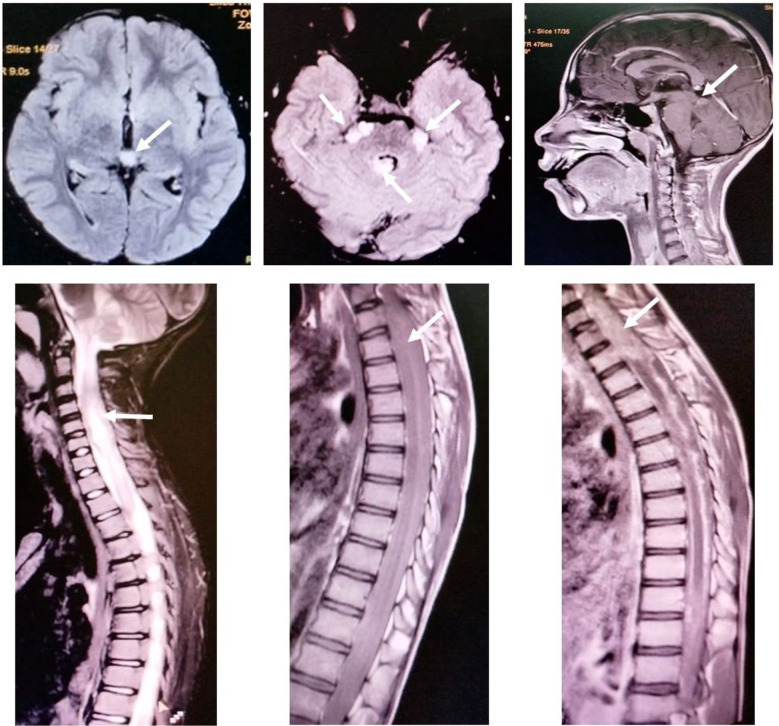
Case 4: MRI Brain and cervical spine with contrast: A 7.7 x 1.4 cm long segment heterogeneous enhancing lesion in the cervicodorsal cord. Multifocal heterogeneous intensity minimally enhancing intracranial extra-axial lesions in the posterior cranial fossa, interpeduncular and quadrigeminal cistern.
